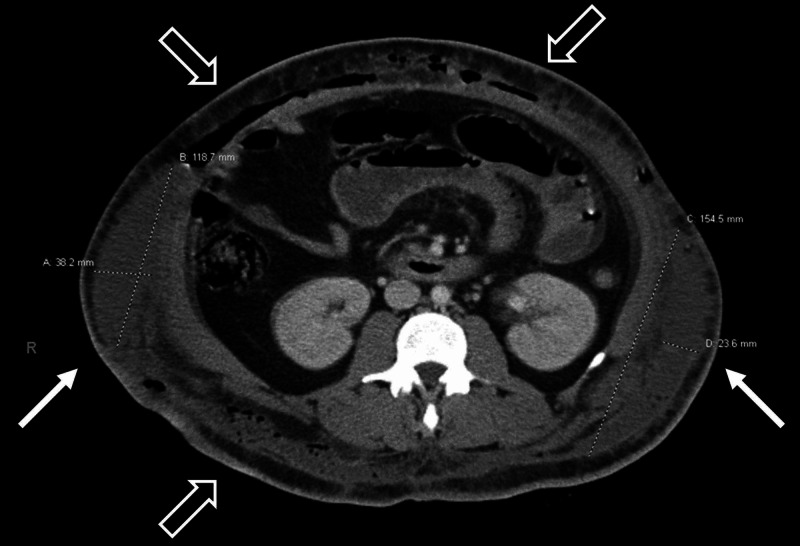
Transverse CT image showing bilateral flank fluid collections (white arrows) measuring approximately 21 x 3.8 x 12cm on the right and 15 x 2.4 x 15.5cm on the left. Numerous foci of subcutaneous emphysema can also be seen (blank arrows).
